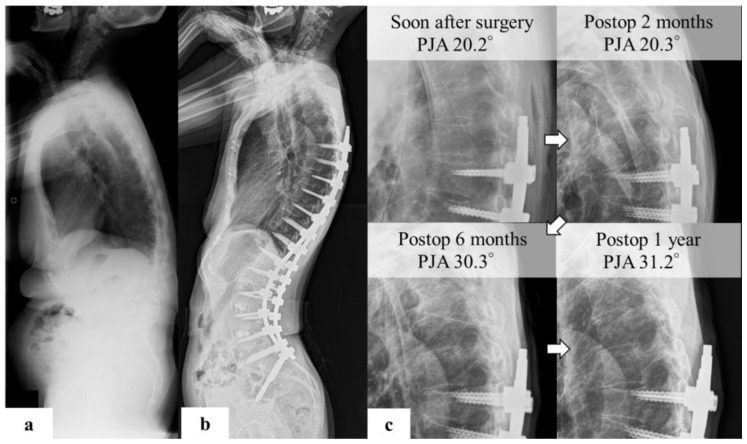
(a) Representative case of PJK preoperative standing X-ray images indicated the following spinal parameters: C7SVA, 86 mm; TK, 20°; LL, 20°; PT, 30°; PI, 53°; TPA, 32°; and PI–LL, 23°. (b) postoperative images show that the PJA was 20.2. (c) PJA developed 6 months postoperatively. The PJA was 31.2° in the first year postoperatively, and the change was 11.2°. This patient complained of implant prominence and pain in the proximal junctional area, but refused reoperation.
